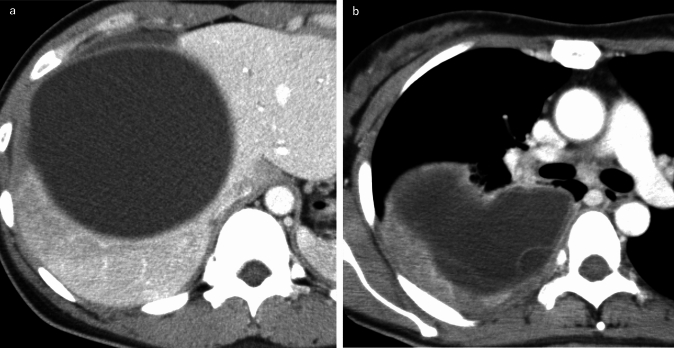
Parasitic embolism—cystic echinococcosis. A woman in her 30 s with a history of residing in the UK presented a year earlier with cough and right-sided chest and abdominal pain. Contrast-enhanced CT revealed a large cystic mass in the patient’s liver (a) and another cystic lesion in the right lower lobe of the lung (b), containing internal septations. Serologic testing confirmed cystic echinococcosis
